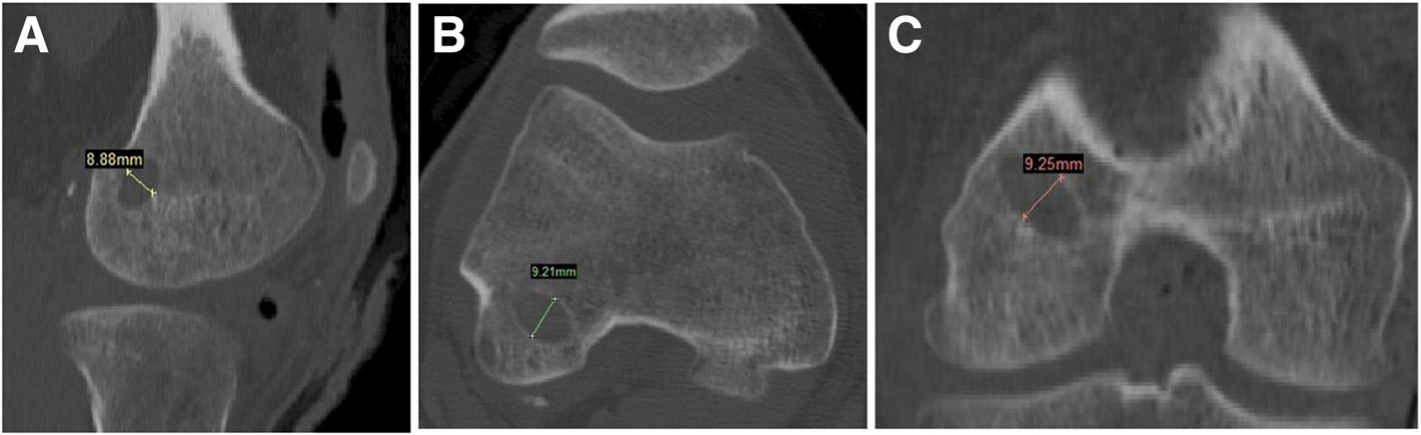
2D CT method. The measurements were made based on the original CT images, and the tunnels were assessed in all three CT planes: coronal (A), sagittal (B), and axial (C).
